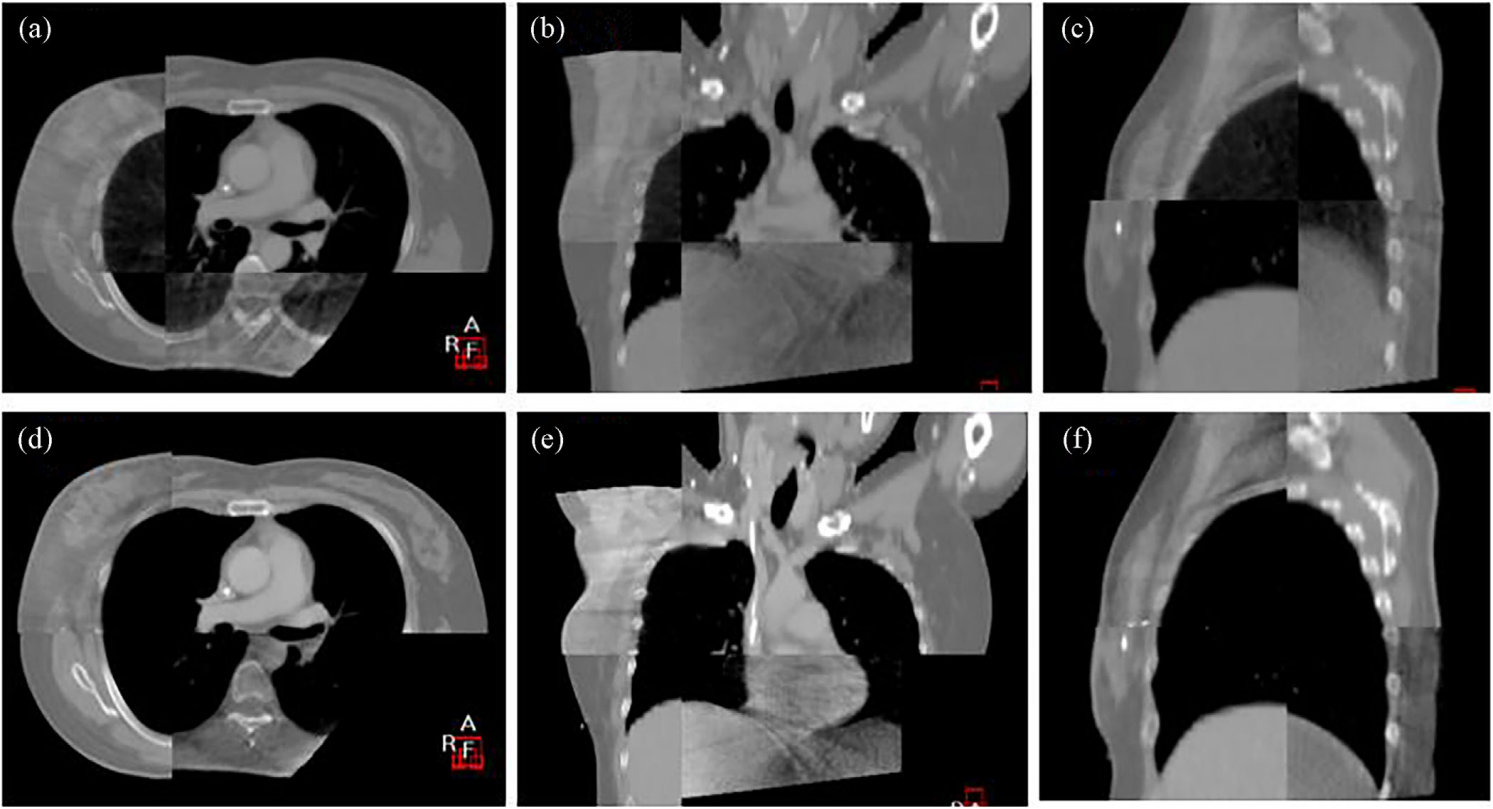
The pictures (a–f) show setup CBCT verification images of a right‐sided breast cancer patient in two different fractions with deep inspiration breath‐hold (DIBH) under the gating points placed at EXP. The transverse plane image (a) shows that insufficient inspiratory volume resulted in excessive setup error in AP direction during treatment, and the sagittal and coronal plane images (b and c) show that insufficient inspiratory volume led to large differences in liver position. However, in another fraction, CBCT images (d–f) show a successful radiotherapy fraction with great DIBH reproducibility.
